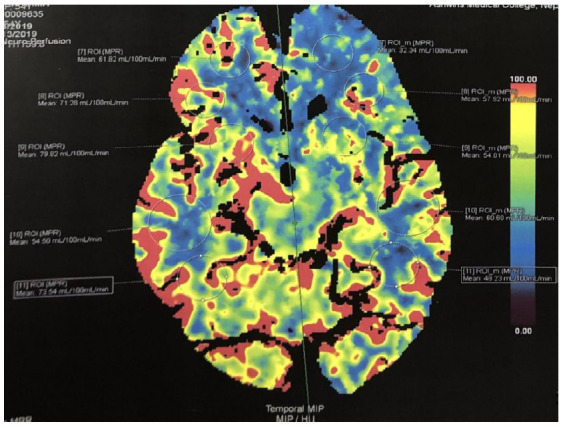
Computed tomography Perfusion showing hypoperfusion on the left ACA-MCA area.
